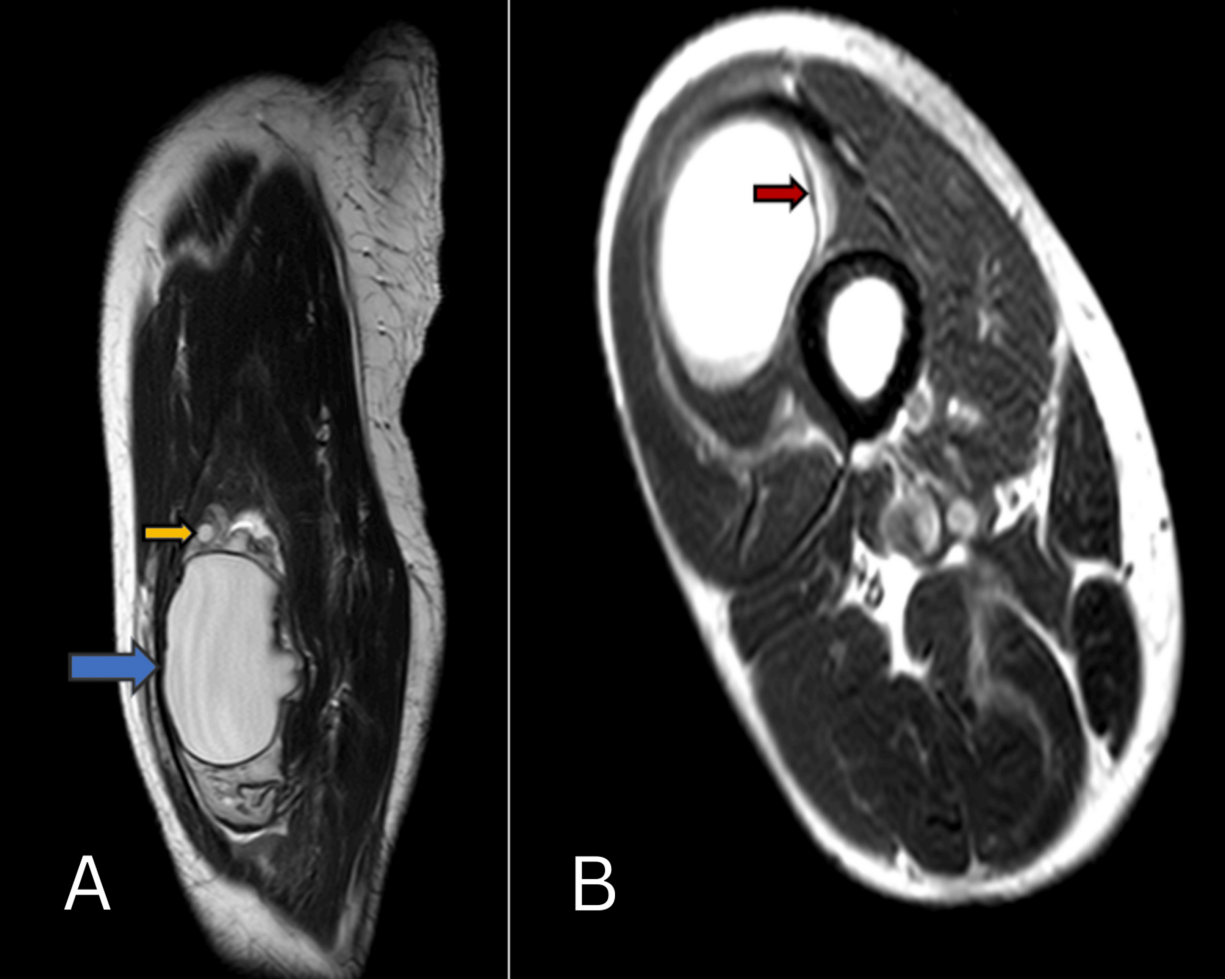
MRI T2-weighted A-sagittal and B-axial images show a well-defined cystic lesion in the vastus intermedius muscle (blue arrow) with a floating membrane within (red arrow) and with small cystic lesions adjacent to it (yellow arrow).
